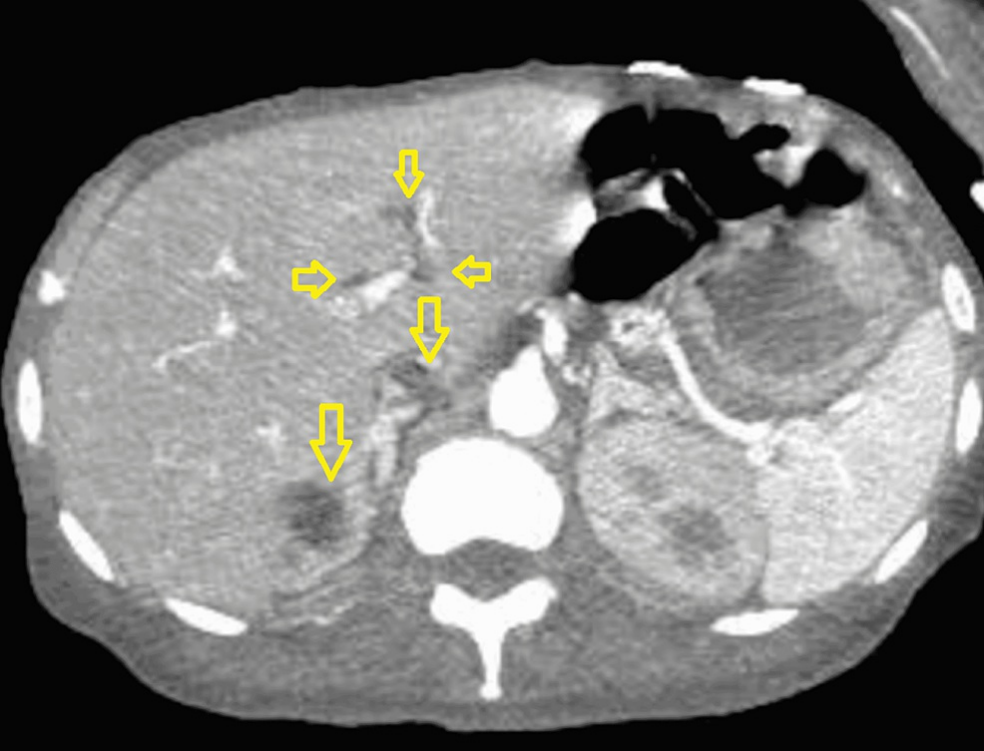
Computed tomography scan of the abdomen showing gas (hypodensities) in the hepatic portal system (arrows) secondary to duodenocaval communication.
